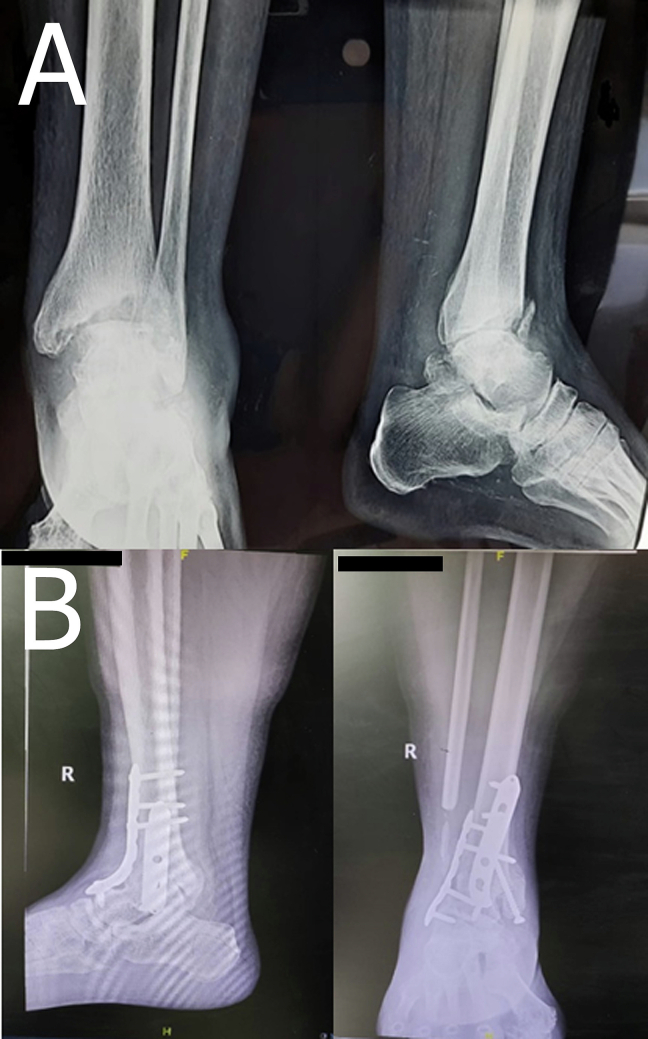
X Ray examinations. (A) Pre-operative right foot Roentgen. (B) Post-operative right foot Roentgen.
